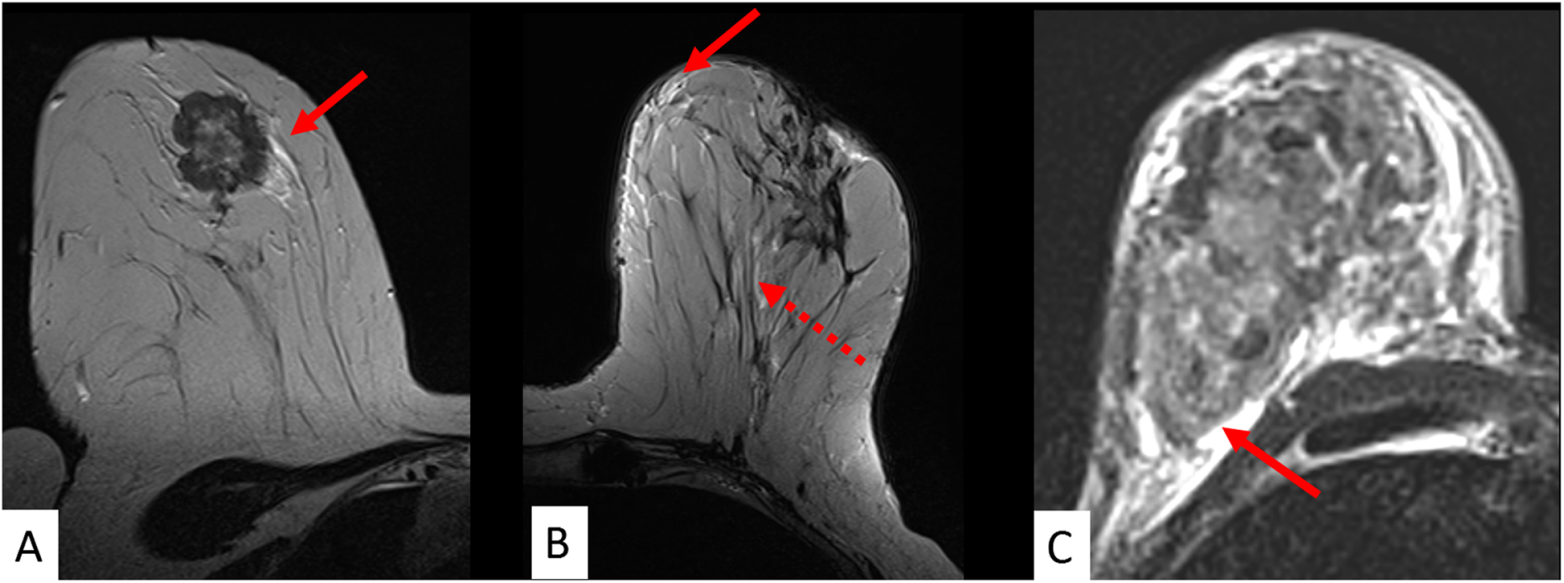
The pivotal role of T2-weighed images: oedema. Any asymmetric ipsilateral T2-weighted signal increase not due to the tumour itself, cysts or artefacts is referred to as “oedema” in A, B, and C. Different oedema patterns are distinguished such as perifocal (A), full red arrow), subcutaneous (B, full red arrow), prepectoral (C, full red arrow), and diffuse (B, dotted red arrow). Oedema is considered among the best evaluated predictive/prognostic criteria and was associated with high grade and nodal-positive cancers as well as disease recurrence (see also Table 1)
